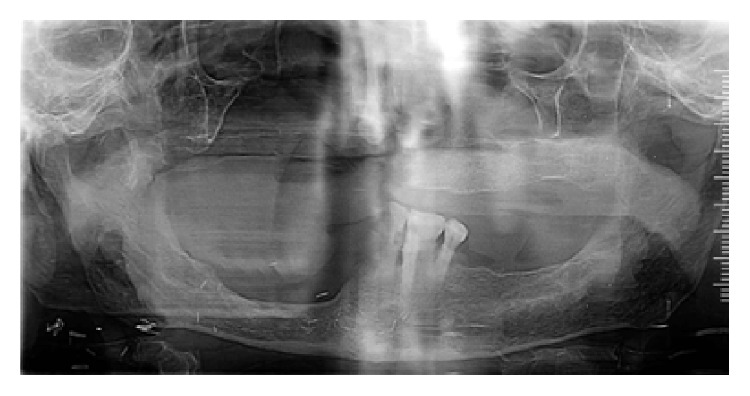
Postsurgical orthopantomograph revealing right side hemimaxillectomy.
